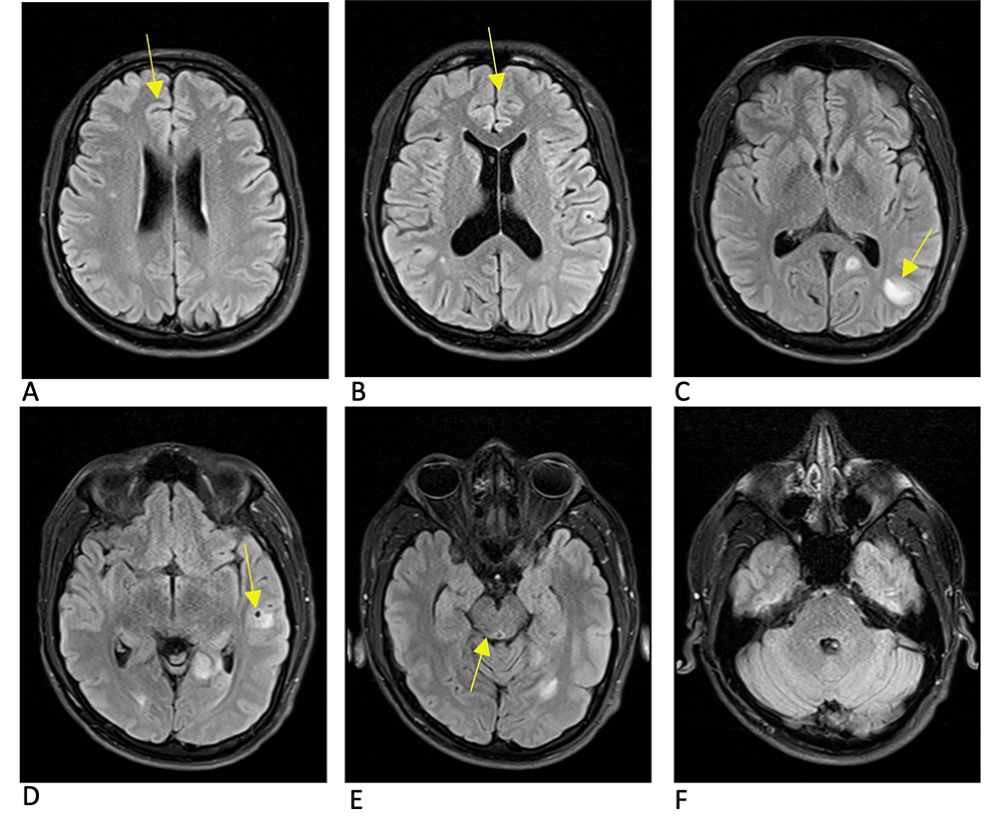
MRI brain T2 FLAIR sequence at the onset of symptoms Multiple bilateral inflammatory lesions are denoted by arrows. The cysts in the anterior right and left frontal lobes (panes A and B) are associated with much less inflammation when compared to the posterior left parietal lesion and the left temporal lesion (panes C and D). The right posterior midbrain lesion demonstrates less T2 fluid-attenuated inversion recovery (FLAIR) signal than would be expected given its size (pane E). There are multiple areas of inflammation seen on T2 FLAIR that do not correlate with a lesion on the T1 post-contrast sequence demonstrating potential additional cysts.
